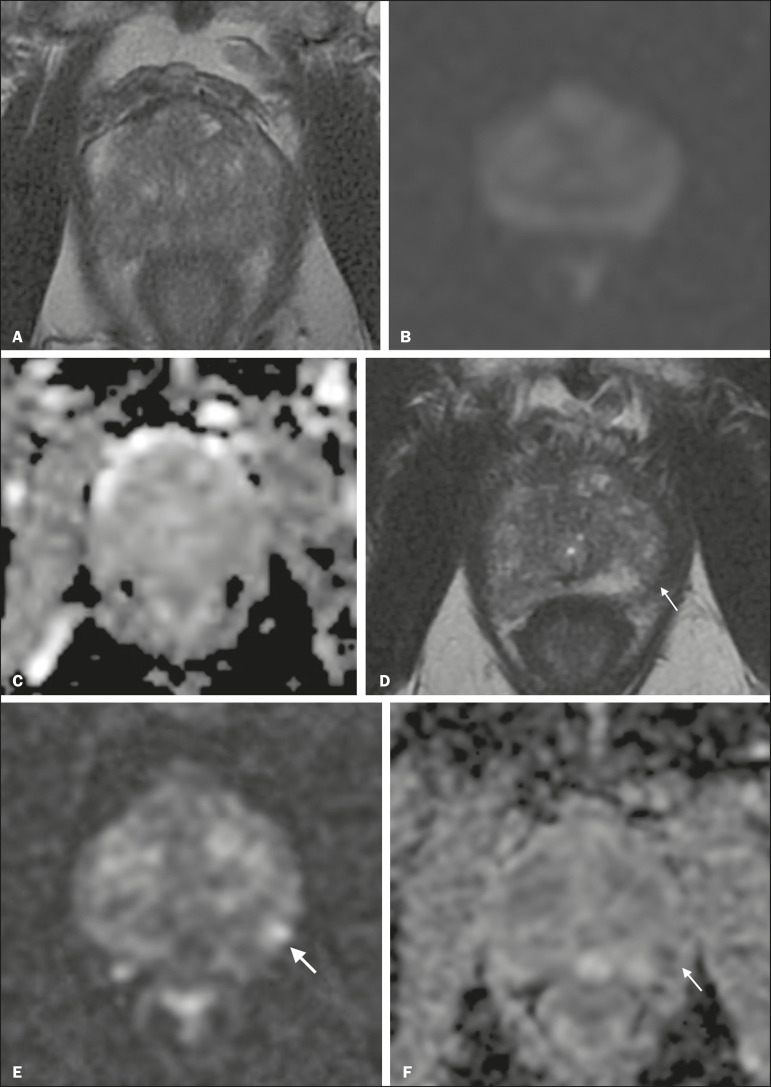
A 54-year-old patient with PCa, classified as International Society of Urological Pathology (ISUP) grade 1 (Gleason score of 6) in 2 of 12 cores in a systematic biopsy, who underwent MRI prior to being enrolled in an AS protocol (in January of 2018). Axial T2-weighted image (A), DWI (B), and ADC map (C), showing no definite lesions. One year later (in January of 2020), another MRI was requested. D: Axial T2-weighted image showing a small but well-defined hypointense area in the left peripheral zone. E: DWI showing a bright area in the same location. F: ADC map showing a small area of restricted diffusion in the same location. A clinically significant lesion was suggested and later confirmed.
